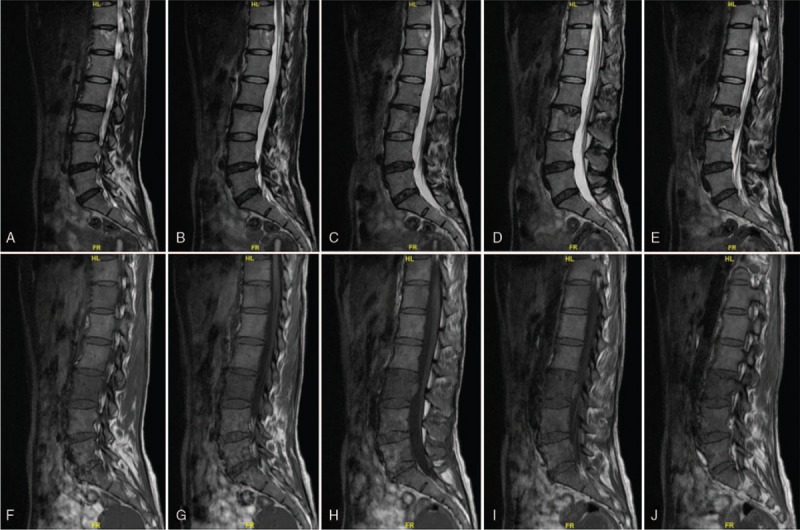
(A–D) Preoperative sagittal MRI scan revealing the density of soft tissues and obvious bony destruction in the T12 and L3, and spinal cord compression caused by thoracic tumor, with increased metastatic marrow infiltration of the vertebra.
